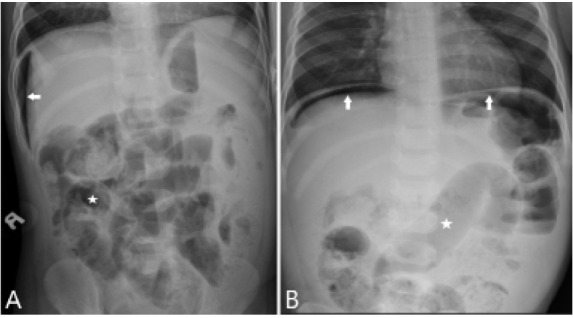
Plain film abdominal radiographs. Panel A (left lateral decubitus view) and Panel B (upright anteroposterior view) showing free air under the diaphragm (arrows) with dilated loops of bowel (stars).
